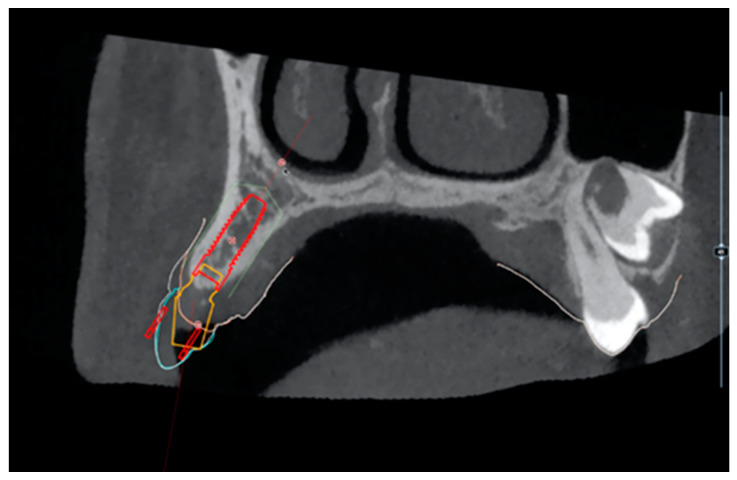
Guided implant planning in zone 1.3 using CBCT, the soft tissue contour, and the digital wax-up. Computer-guided planning of abutment angulation was made according to the digital diagnostic wax-up of the 1.3 element.
